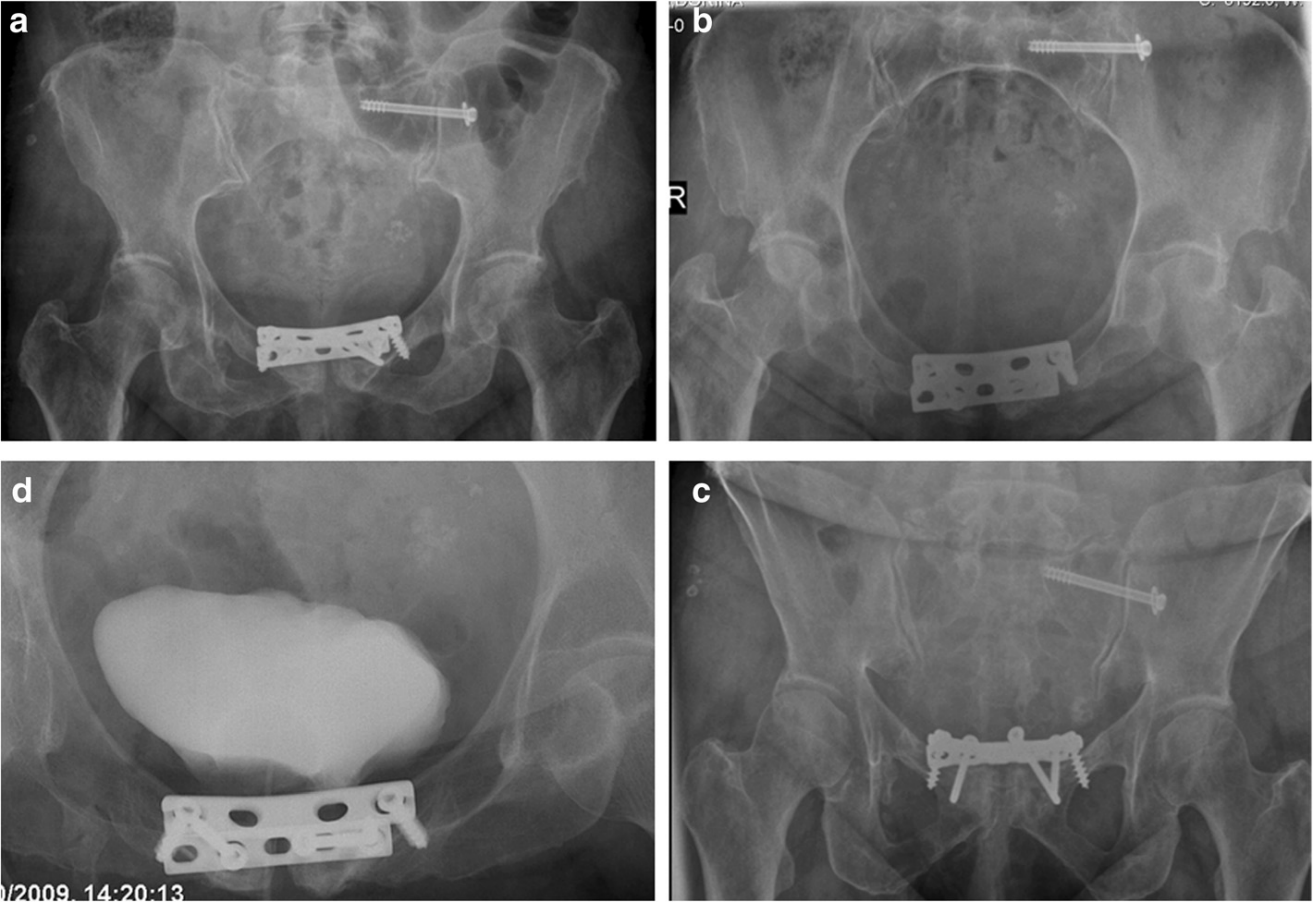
Pelvic ring x-rays and a cystogram after repeated screw expulsion. Pelvic ring images AP [a] inlet [b] and outlet [c] views, obtained after the expulsion of the second screw (October 2009). The three projections show five screws remaining compared to the seven screws which had been placed initially (compare with Fig. 2). Two 6.5 mm cancellous screws are present. All five remaining screws have loosened as seen in the three views. The position of the washer on the S1 iliosacral screw has changed and is no longer flush to the outer iliac cortex. These changes indicate that the 7.3 mm screw has also loosened when compared to its original position. A cystogram view [d] shows the contrast material adjacent to the posterior 4.5 mm DCPlate with its two 6.5 cancellous screws
